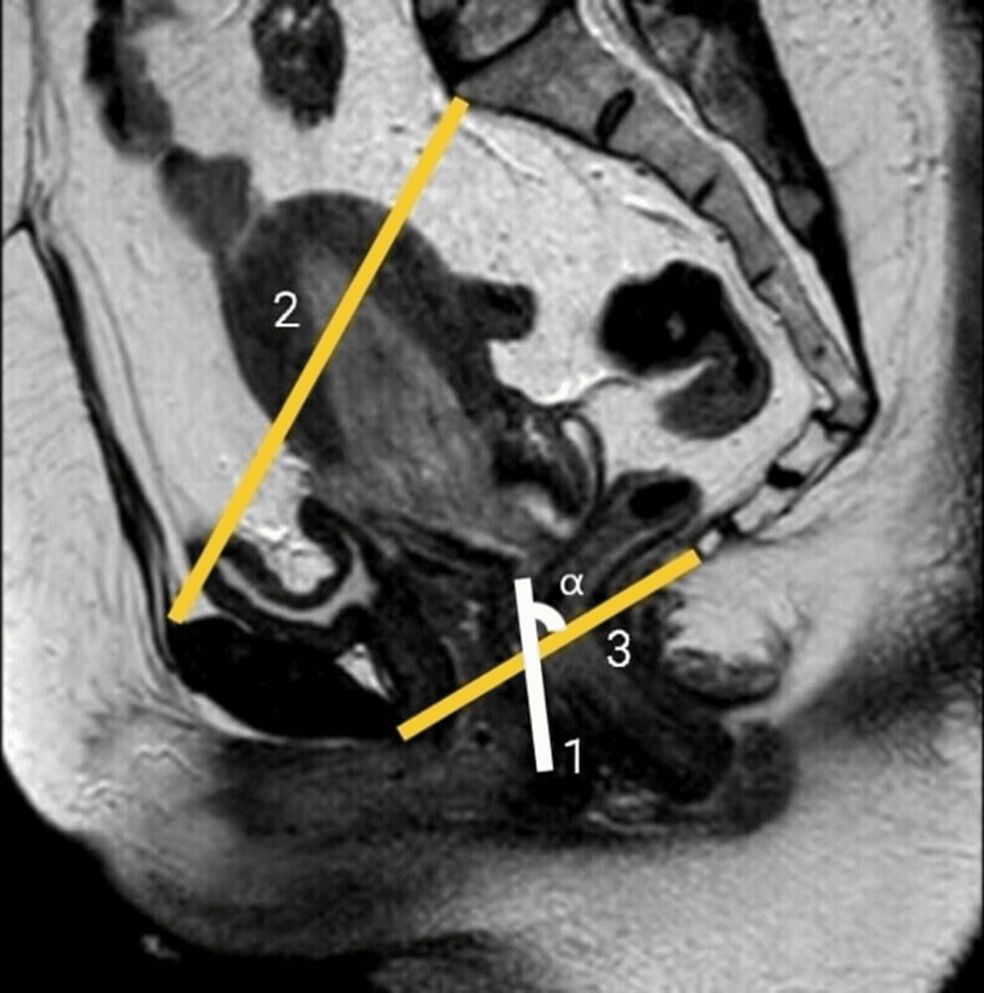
T2-weighted TSE sagittal MRI image of the normal cis-female Original MRI image of a cis-female patient taken from the archive. 1 - Neovaginal depth; 2 - Inlet of the pelvis (IOP); 3 - Inferior pelvic aperture (IPA); α - Alpha angle
